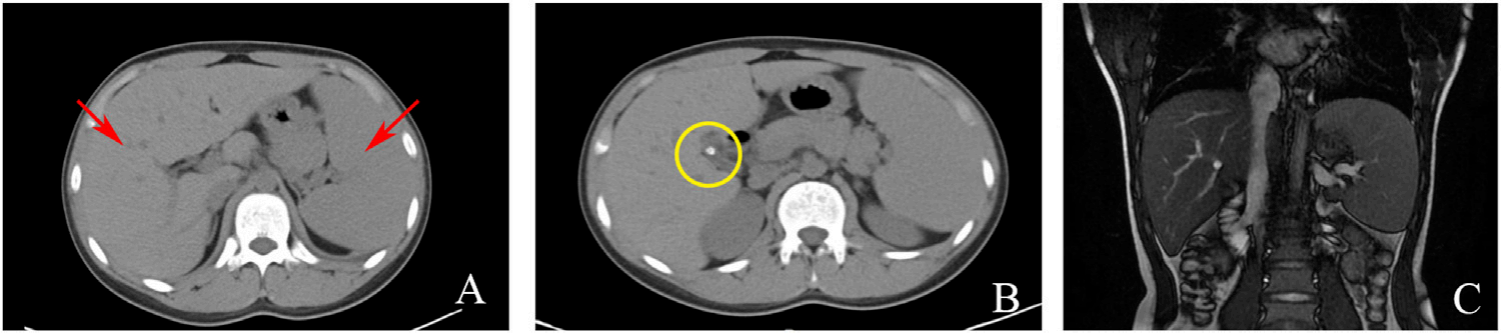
Imaging examinations: (A) CT scan showing liver and spleen enlargement (red arrow); (B) CT scan showing gallbladder neck stones (yellow circle); (C). MRCP showing a normal intrahepatic and extrahepatic biliary tree and pancreatic ductal system.
